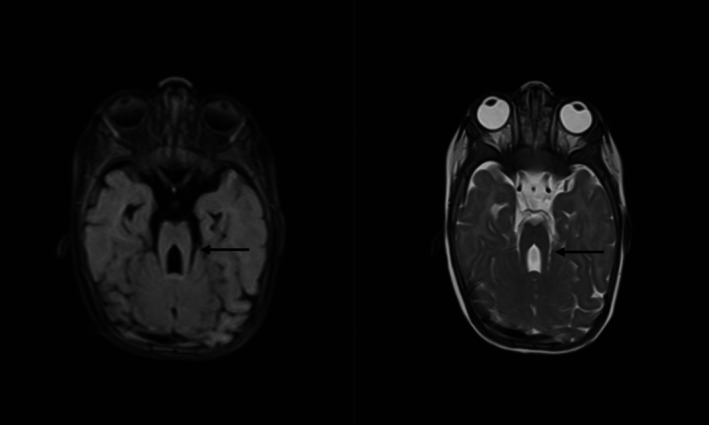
Brain MRI findings were consistent with Joubert syndrome, demonstrating the characteristic ‘molar tooth sig’, which arises from cerebellar vermis hypoplasia, elongated superior cerebellar peduncles, and a deep interpeduncular cistern. This radiological hallmark supported the clinical diagnosis of Joubert syndrome in our patient.
